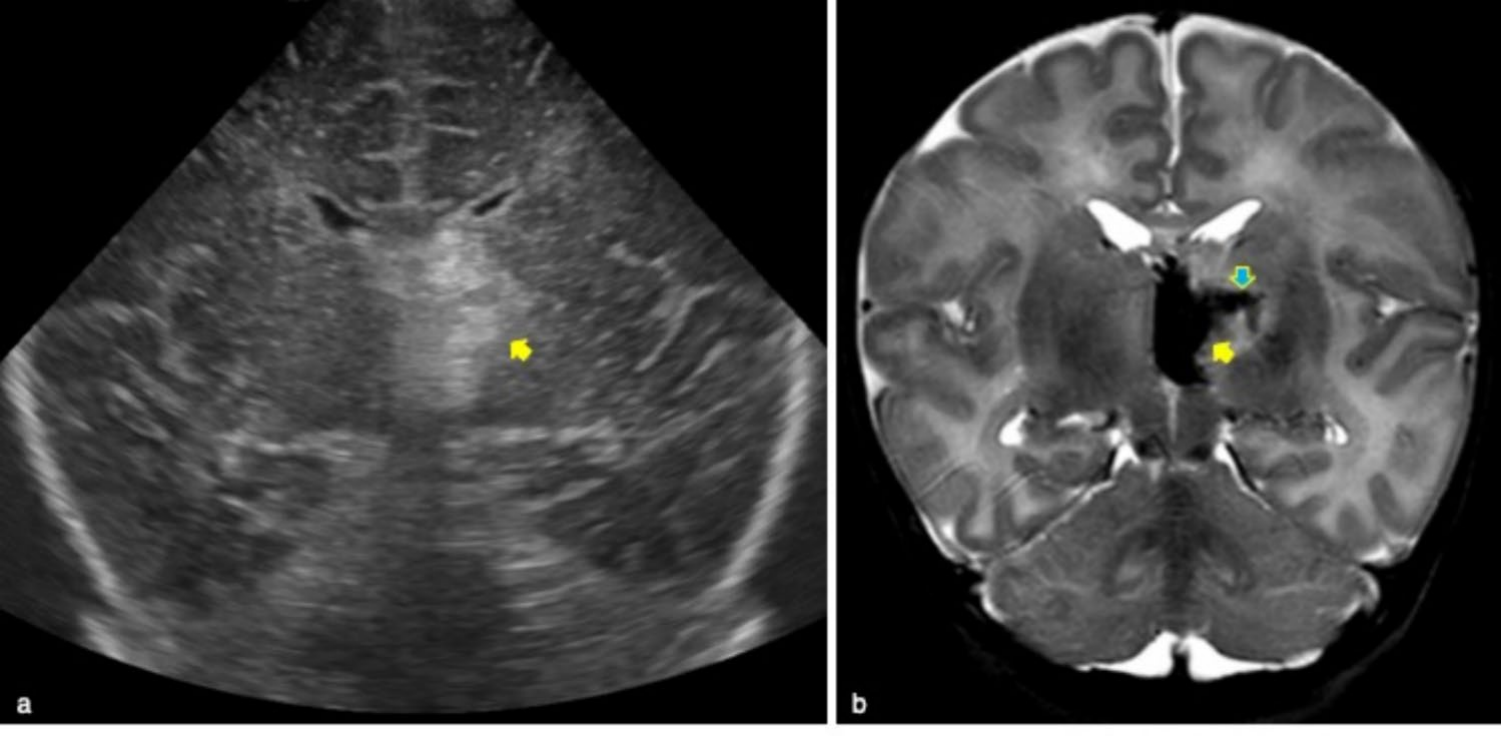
Full term female who underwent radiological examinations at four day of life for hypothermia. Cerebral ultrasound showed left unilateral thalamic echogenic area on coronal plane (yellow arrow in a). MR well demonstrated left thalamic hemorrhagic stroke on coronal T2w image (yellow arrow in b) associated with linear hypointense area corresponding to thrombosis of thalamic vein (blue arrow in b)
